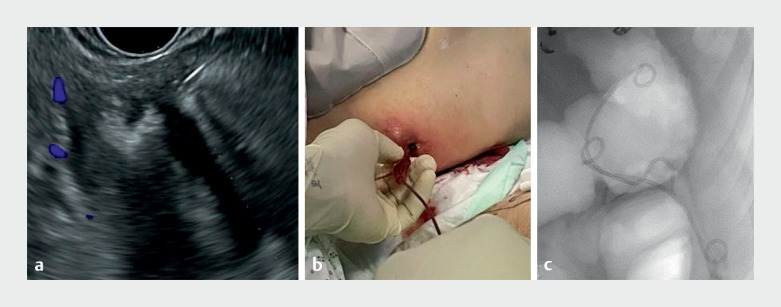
Endoscopic ultrasound (EUS)-guided drainage of a pancreatocutaneous fistula after acute pancreatitis.
